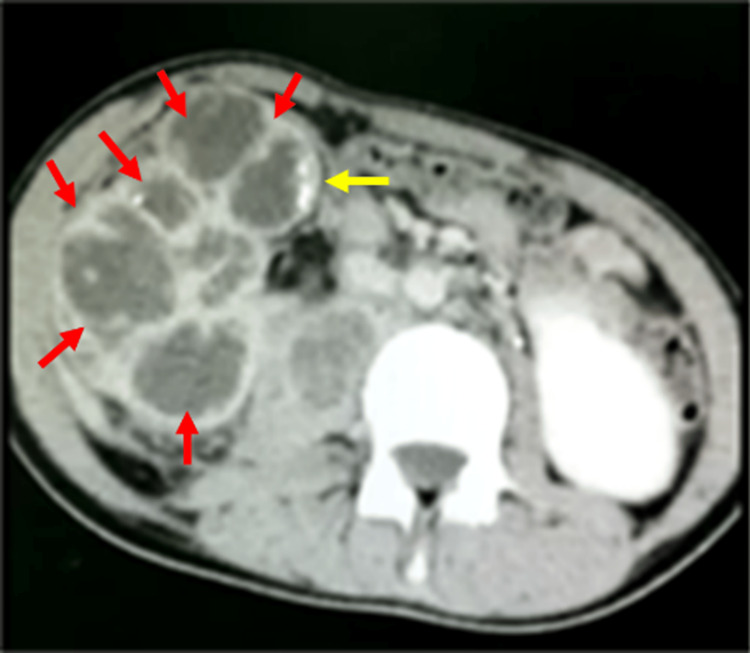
Computed tomography of the right kidney Red arrows: Kidney cysts; Yellow arrows: Calcifications
